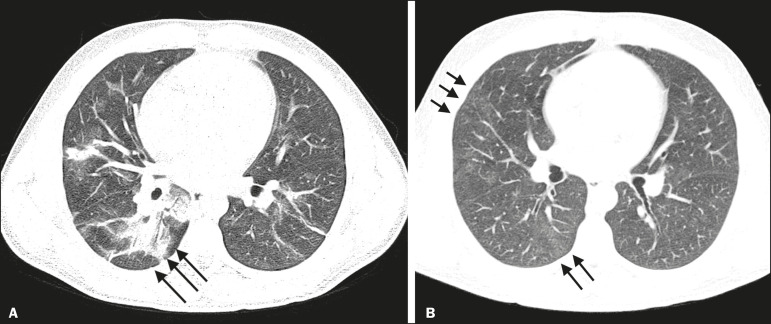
A 29-year-old male patient. A: CT scan acquired on day 4 after symptom onset, showing peribronchovascular consolidations (a stage 3 finding) in the right lower lobe (arrows). B: CT scan acquired on day 14 after symptom onset. The patient still had a mild cough and dyspnea. There are discrete residual ground-glass opacities (a stage 4 finding) in the right lung (arrows).
